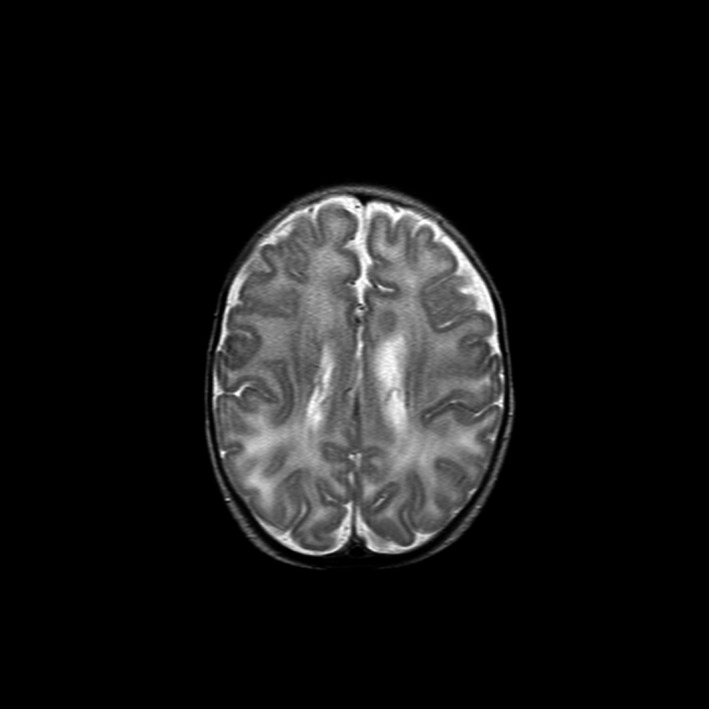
Coronal section of a T2 weighted MRI of the patient's head demonstrating a bulky choroid plexus on the left
